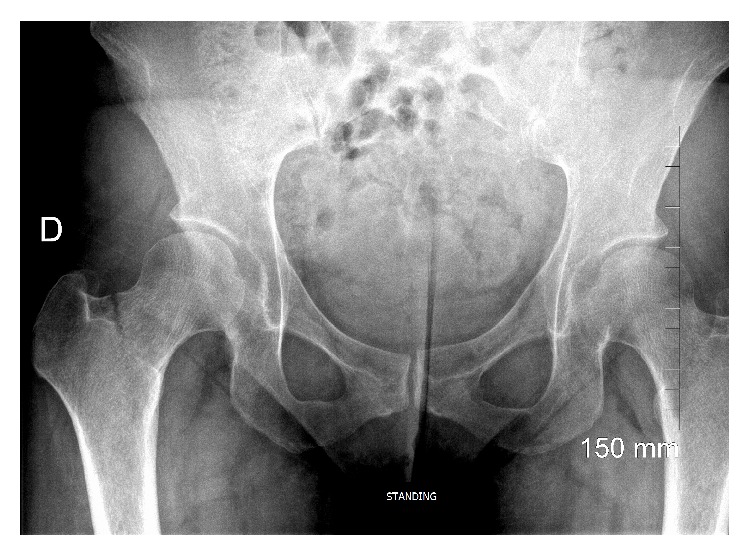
Pelvic X-ray, anteroposterior view. Basicervical fracture of the right femur.
